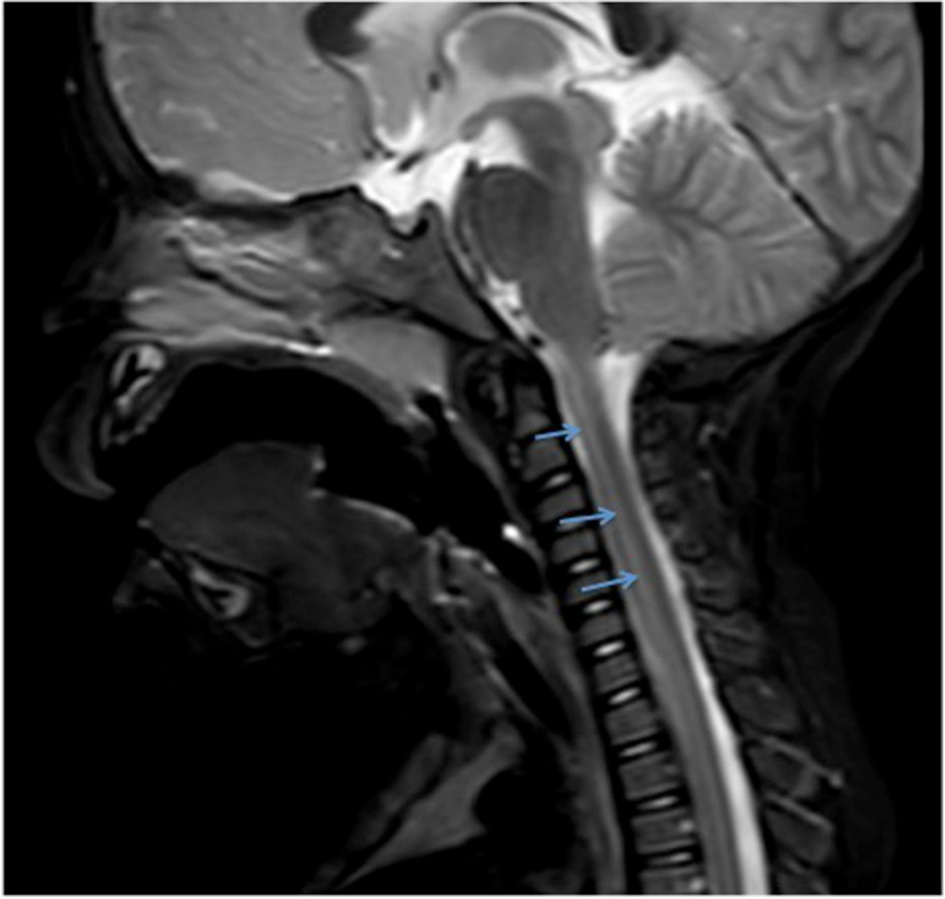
T2-weighted sagittal MRI noting a normal central canal (arrows).
